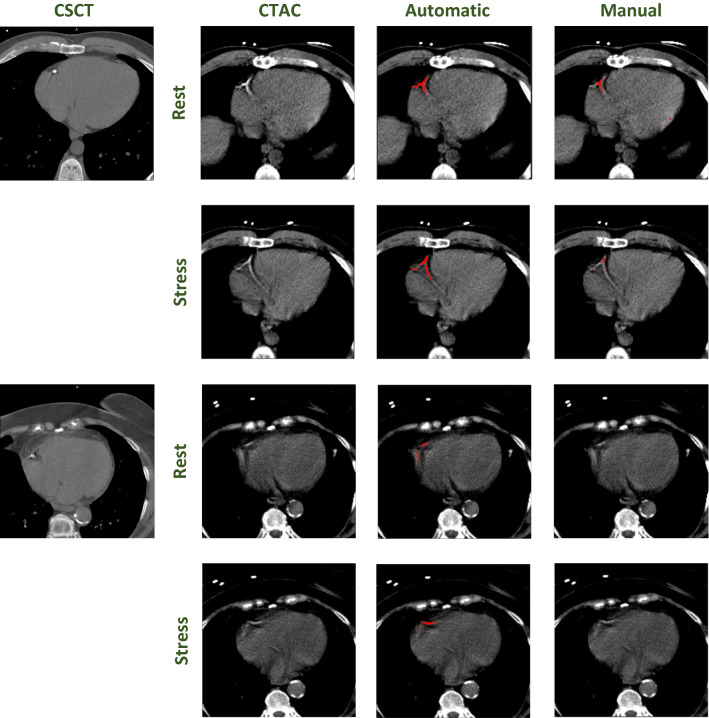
Examples of pairs of CSCT, rest and stress CTAC with CAC lesions. Overlays (red) are shown on the CTAC scans for the automatic method that is independent of the CT-number threshold and manual calcium scoring, which uses the 130 HU threshold
